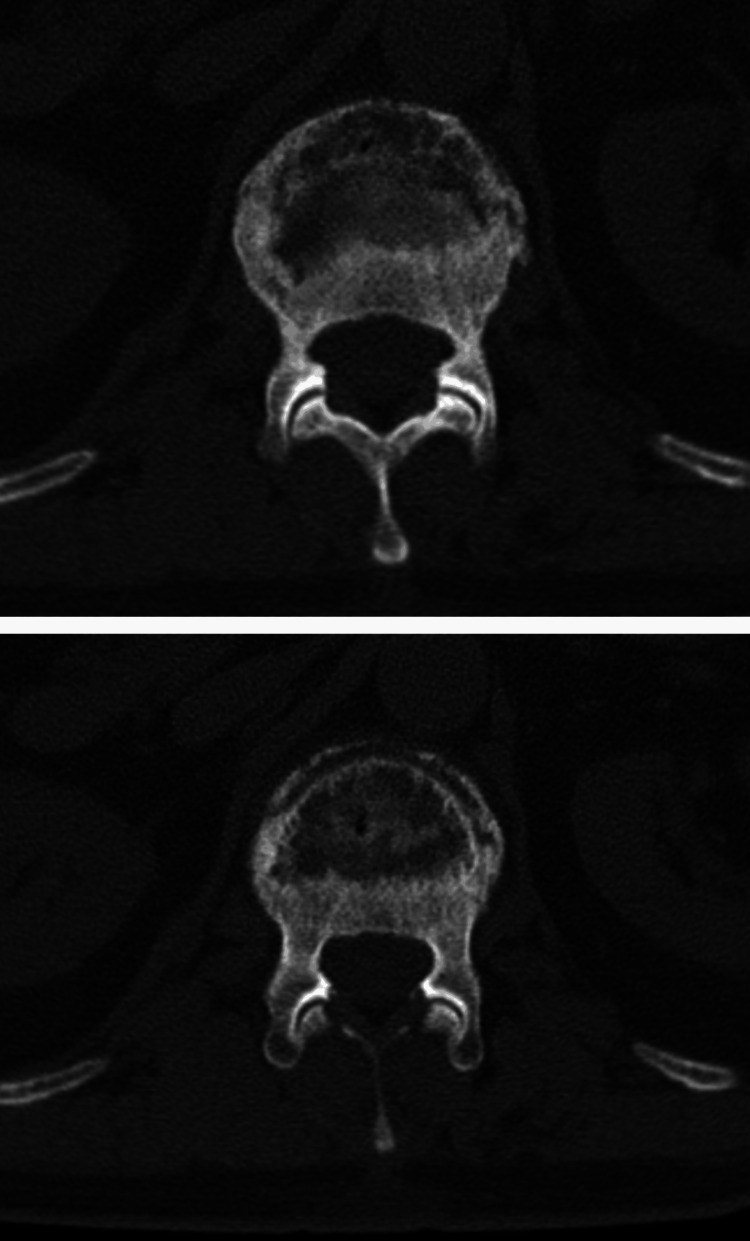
Axial (transverse) CT scan view showing L1 vertebral fracture.
